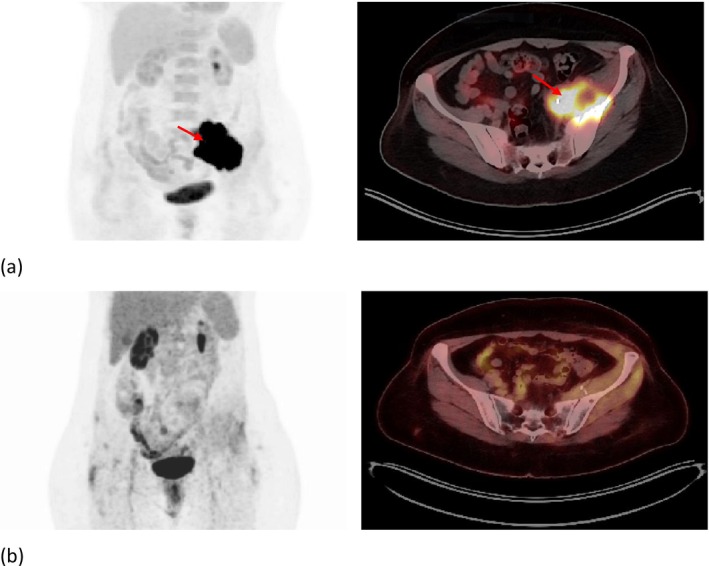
(a) pre‐treatment PET‐CT scan showing A soft tissue mass measuring 75 × 65 mm with SUV_max_ of 32.2 and central hypoactivity in the left hemipelvis region involving the left psoas, left iliac (and apparently left gluteus medius) muscles, and the left iliac bone wing (red arrow), (b) post‐treatment PET CT scan showing complete response, with no residual mass and no apparant abnormal FDG uptake.
